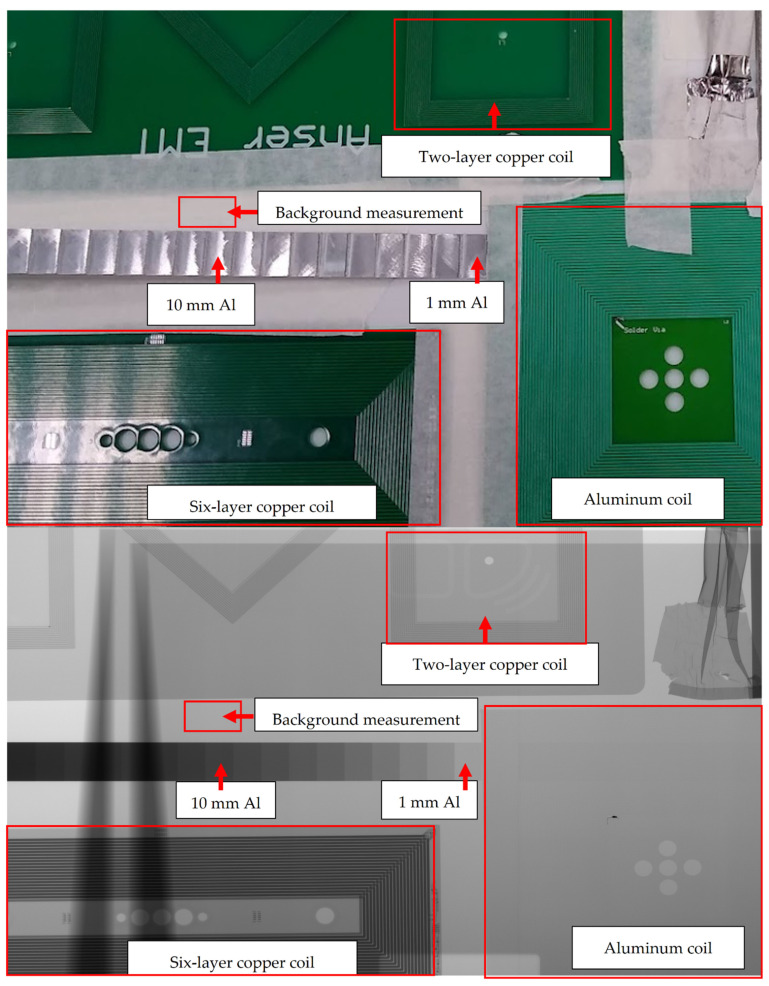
X-ray analysis of the 3 different coil designs with a 1–20 mm aluminum step wedge for reference.
